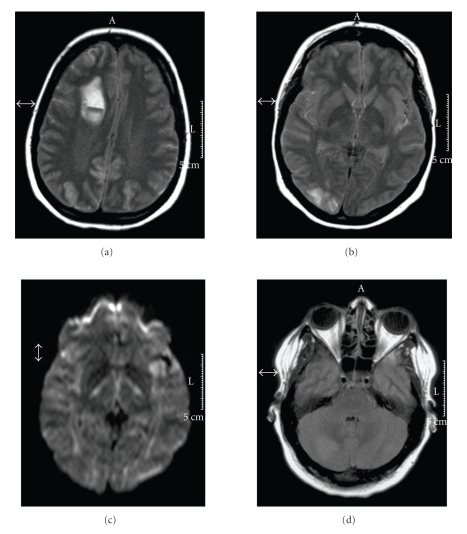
Axial FLAIR sequences show an ICH in the right frontal lobe (a). The combination of hyperintensities rostral of the ICH and in the right occipital lobe (b) with a normal DWI (c) in these areas is consistent with a vasogenic edema. The alterations in the cerebellum were largely unchanged (d).
